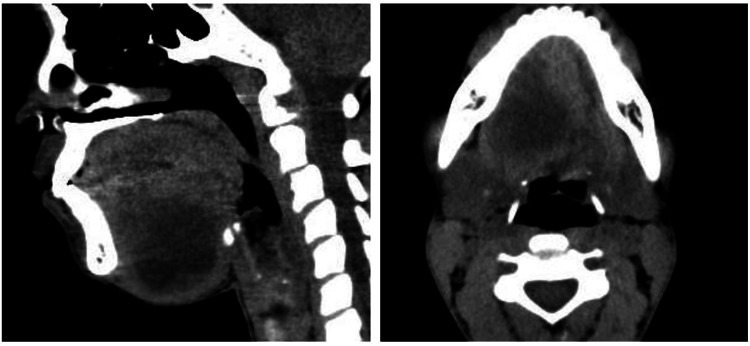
Computed tomography images of the neck Computed tomography images in sagittal (left-hand side image) and axial view (right-hand side image) show a homogeneous, well-defined lesion in the sublingual floor of the mouth region.
